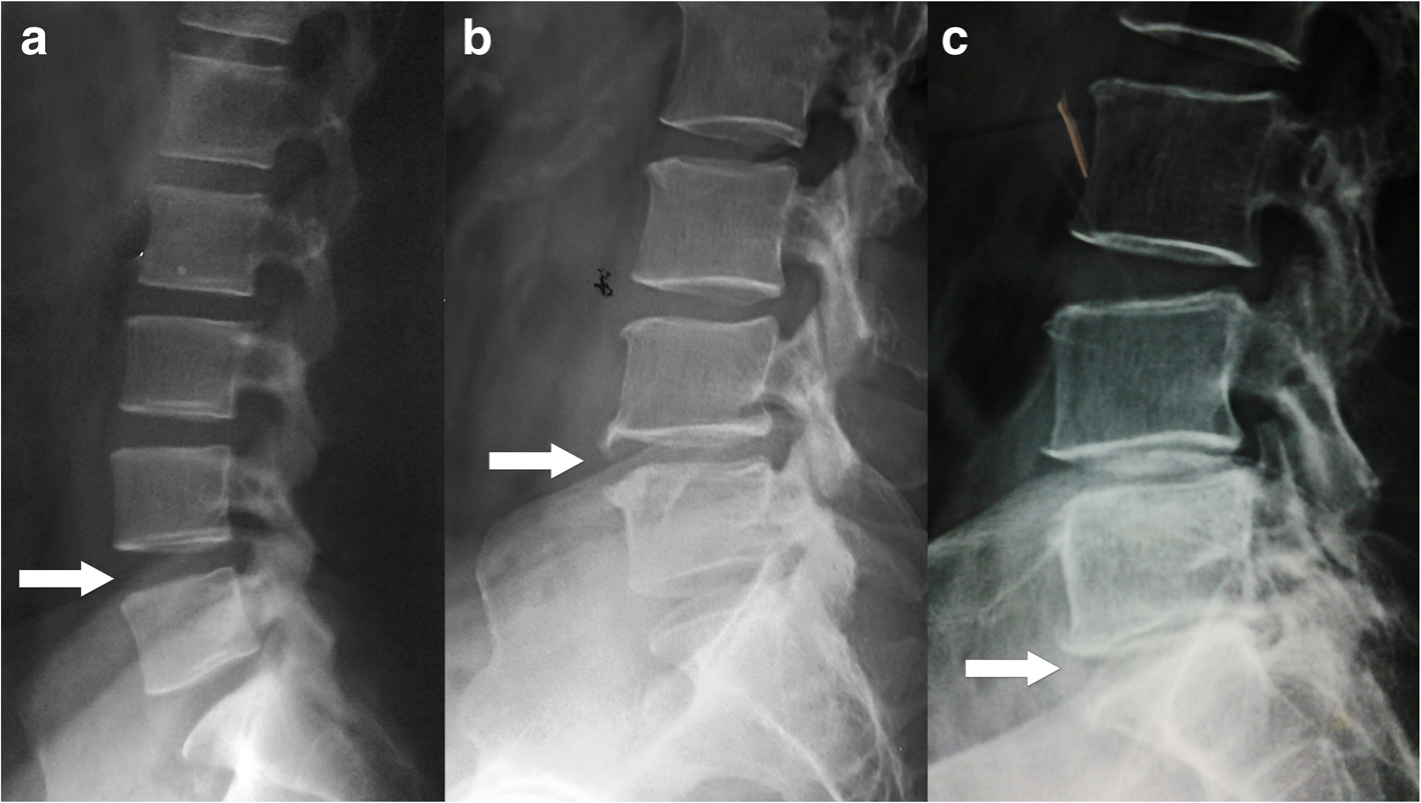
Assesment of the x-ray features of lumbar disc degeneration - lateral x-ray of lumbar spine. Arrows - a – no disc space narrowing/anterior osteophyte (grade 0 lumbar disc degeneration), b – mild disc space narrowing and small anterior osteophyte, (grade 1 lumbar disc degeneration) c – moderate disc space narrowing and small anterior osteophyte (grade 2 lumbar disc degeneration)
